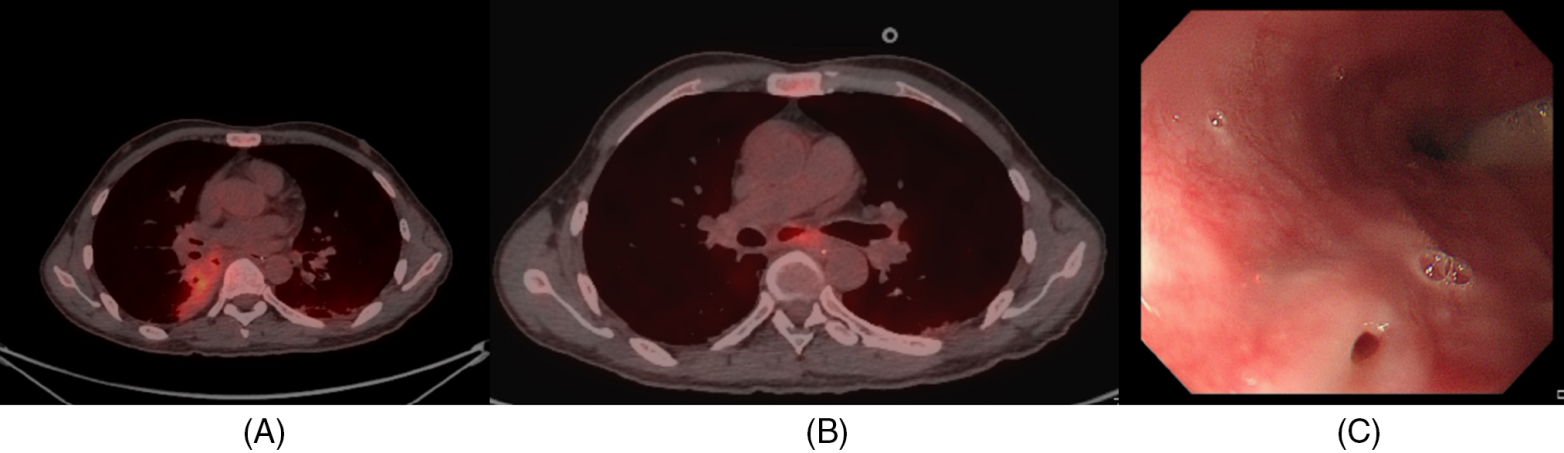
(A) positron emission tomography‐computed tomography before antifungal treatment demonstrated a patchy high‐density shadow in the right lower lobe with indistinct borders, accompanied by a cavity formation with an SUVmax of 6.7 and accompanied by multiple cystic lucent shadows; (B) a cystic lucent shadow was seen beneath the carina, with a slightly increased fluorodeoxyglucose uptake at the margins, yielding an SUVmax of 4.0, which was partially connected to the adjacent oesophagus, with multiple enlarged lymph nodes in the mediastinum, yielding; (C) gastroscopic image of the oesophageal mucosa (30 cm from the incisors) demonstrating an oesophagomediastinal fistula.
